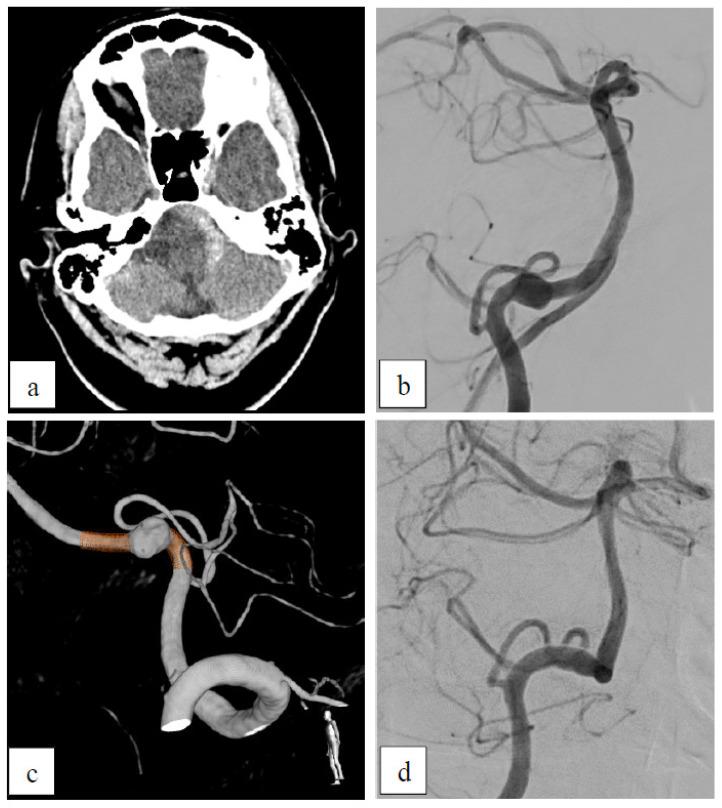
Dissecting aneurysm. (a) Native CT scan showing SAH located around the medulla oblongata and the left cerebellar hemisphere. (b) Angiogram of the left VA shows the dissecting aneurysm of the V4 segment. (c) 3D-angiogram with planned positioning of the FDS device. (d) In the FU, after 6 months, there was complete occlusion of the aneurysm.
